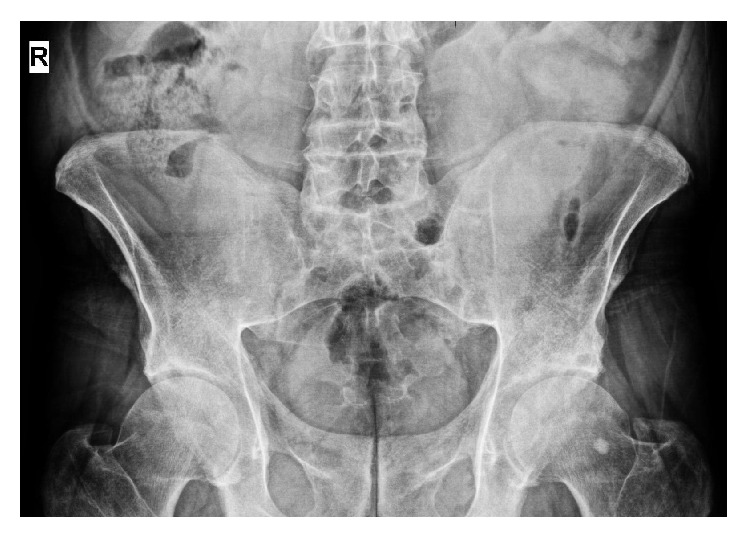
Pelvic X-ray showing bilateral grade III-IV sacroiliitis.
